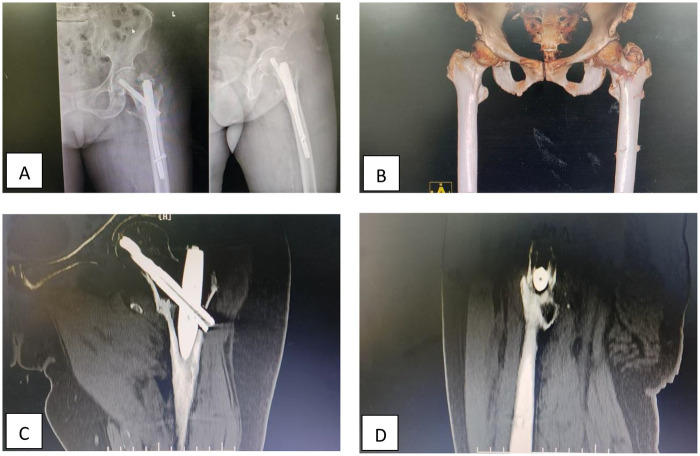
Postoperative three-day follow-up x-ray (A) and 3D CT (B) postoperative three-month anteroposterior (C) and lateral (D) CT views.
